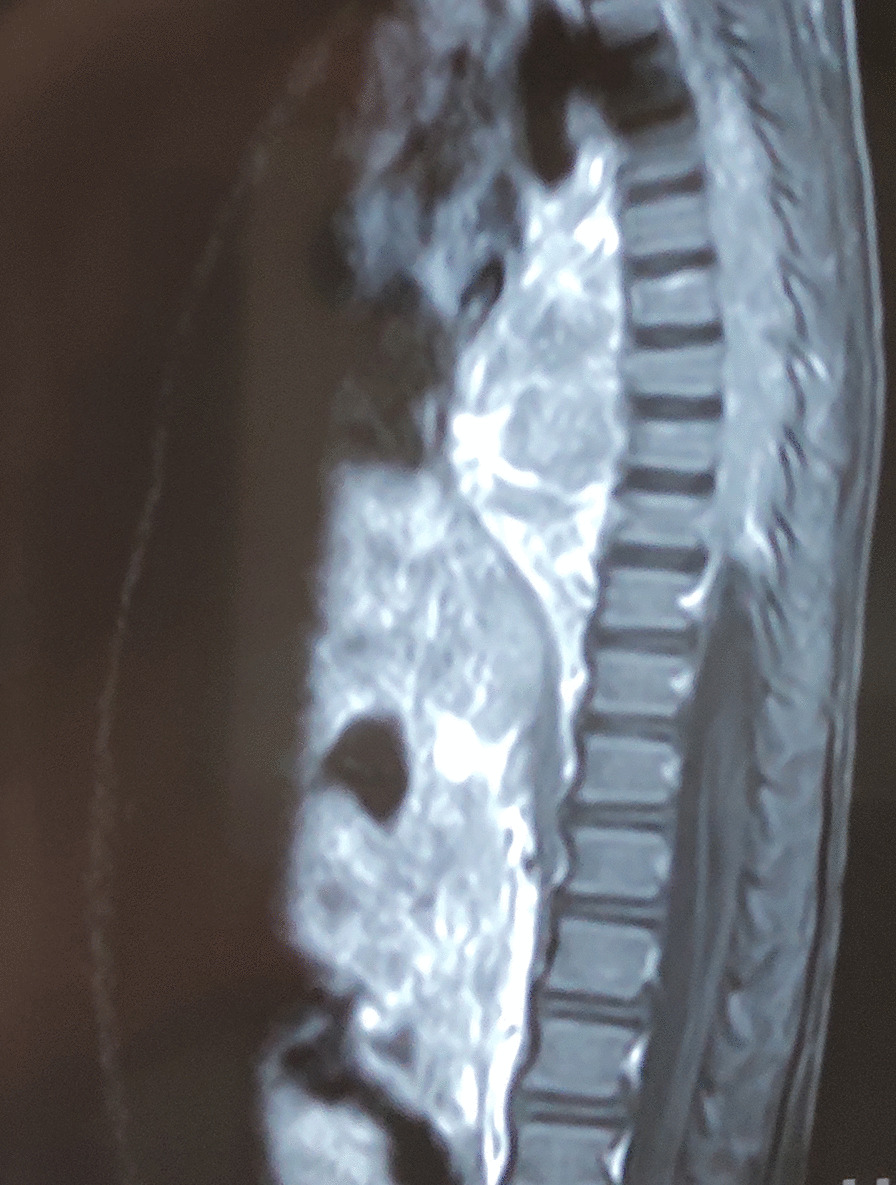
Magnetic resonance imaging showing the tumor was located in right posterior mediastinum, paravertebral space and canalis vertebralis
